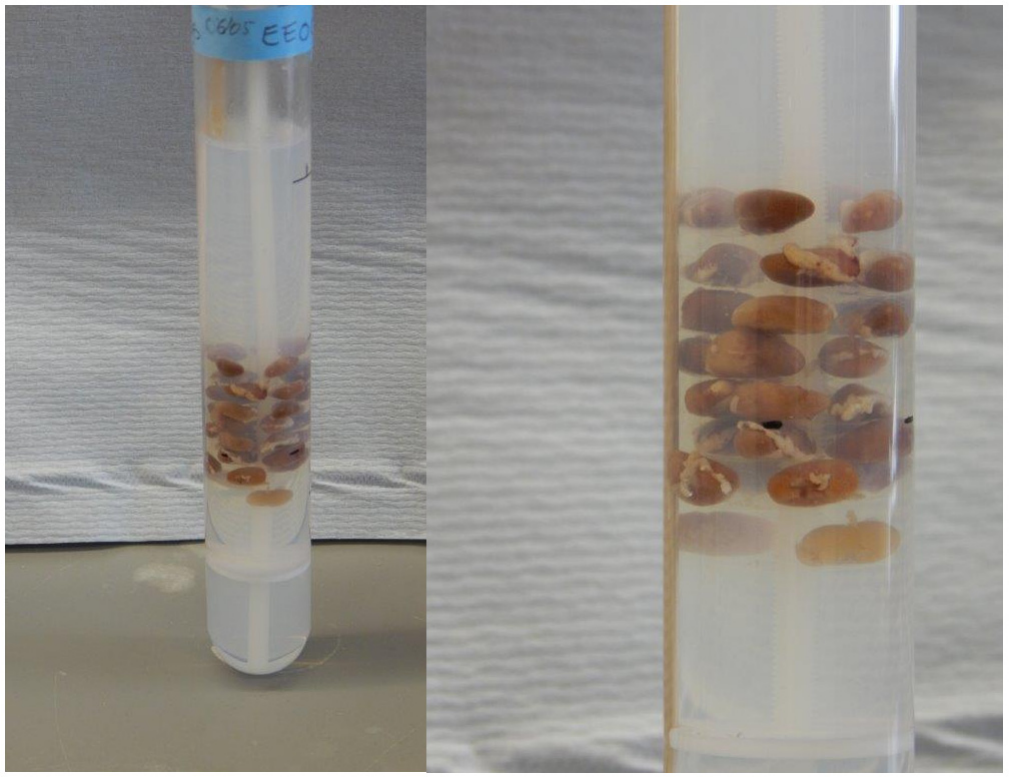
Scanning fixed organs after agar embedding. Up to thirty-two paraformaldehyde-fixed kidneys can be placed in eight layers of four kidneys, and embedded in agarose containing the contrast agent. The tube is then subjected to MR imaging.
